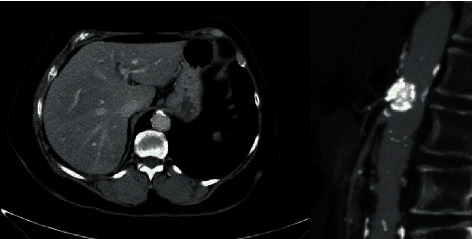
CT lateral view shows a severely calcified origin of the coeliac trunk and significant stenosis in the origin of the superior mesenteric artery. Axial image shows a thrombus inside the calcification (case 1).
